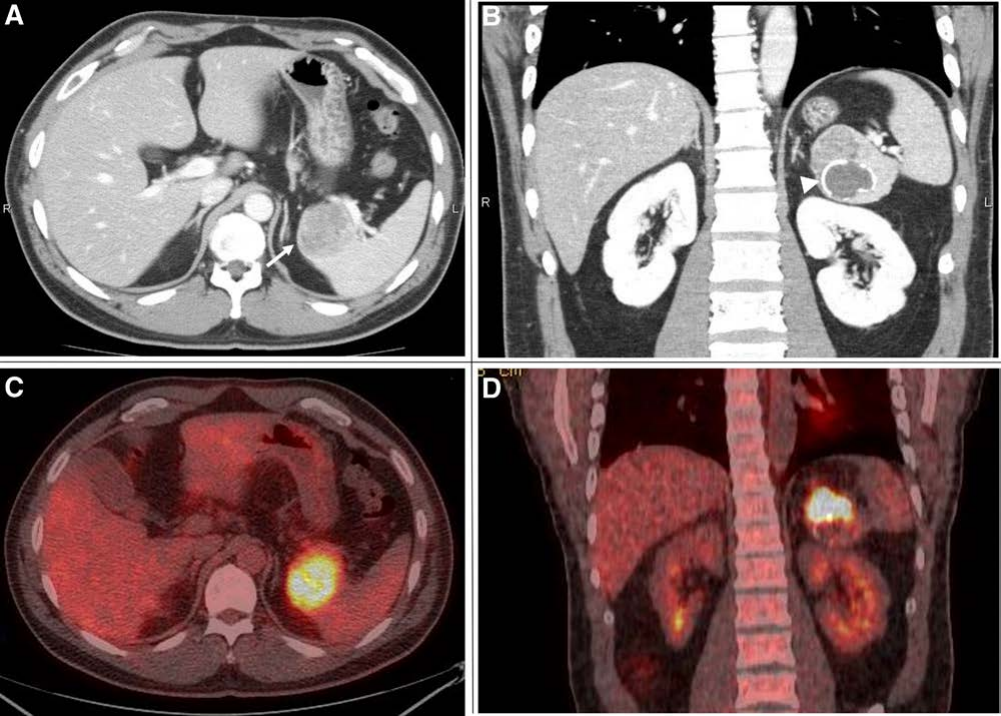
Preoperative CT and FDG-PET CT picture. (A) Computed tomography (CT) demonstrated a low attenuation solid mass protruding from the lower pole of the spleen (white arrow). (B) A cystic component with mural calcifications adjacent to the mass was shown. (arrow head). (C and D) The tumor was FDG avid on FDG-PET/CT. FDG-PET/CT = fluorodeoxyglucose-positron emission tomography.
